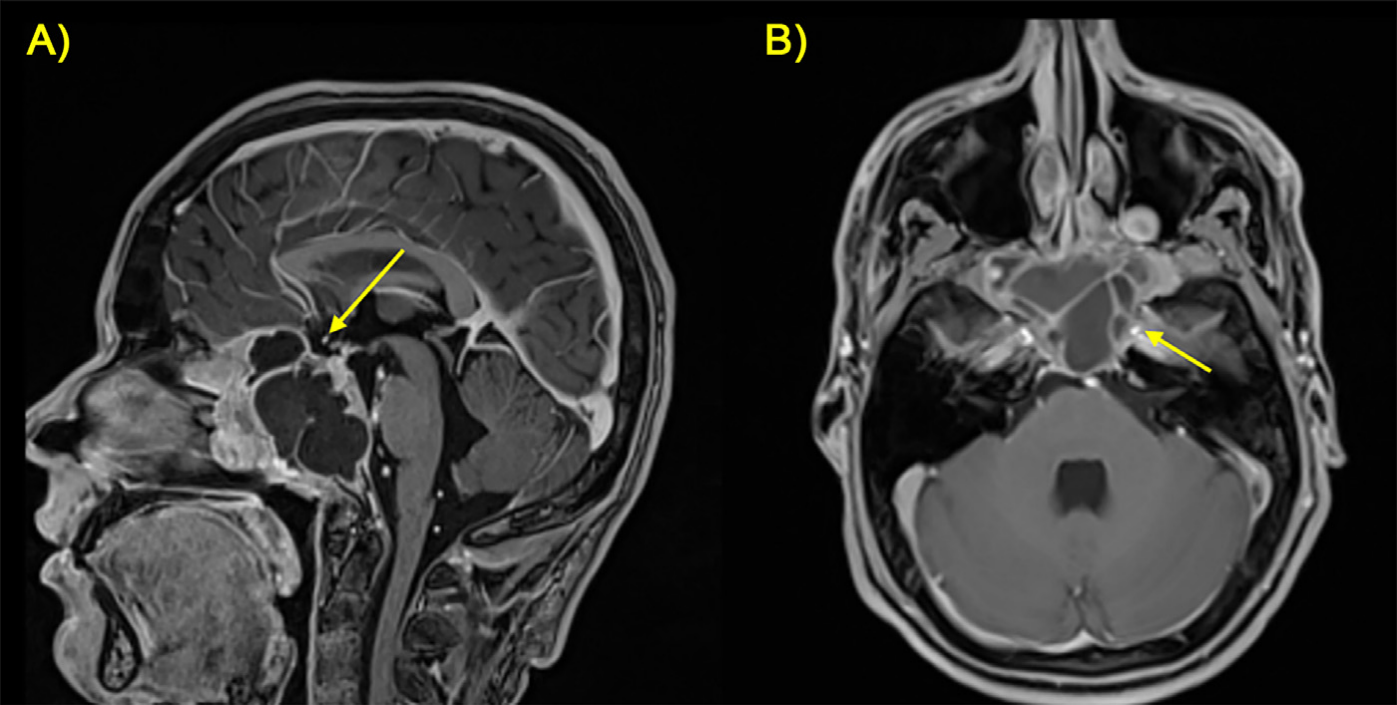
(A, B) Coronal (A) and axial (B) sequences post-contrast administration performed on 3T MRI equipment showed mostly peripherical enhancement of the mass (yellow arrow).
